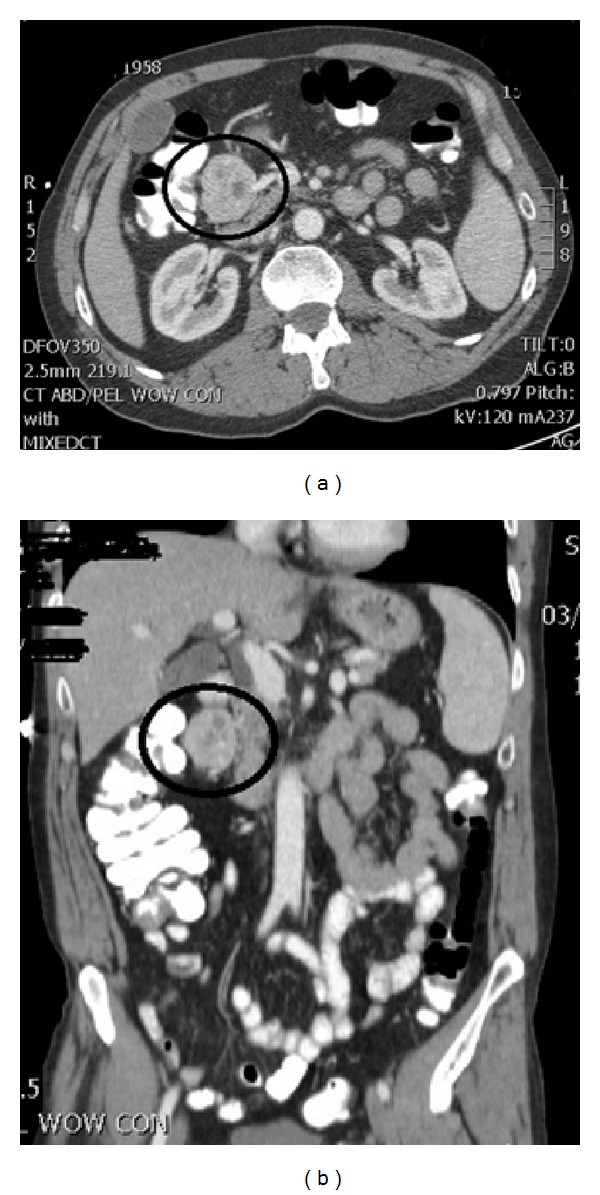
CT scan of abdomen and pelvis with contrast in (a) axial view and (b) coronal view showed well-defined 4.5 cm enhancing mass of mesocolon which was located anterior to descending duodenum, posterior to gastric antrum, and just medial to the hepatic flexure of colon.
